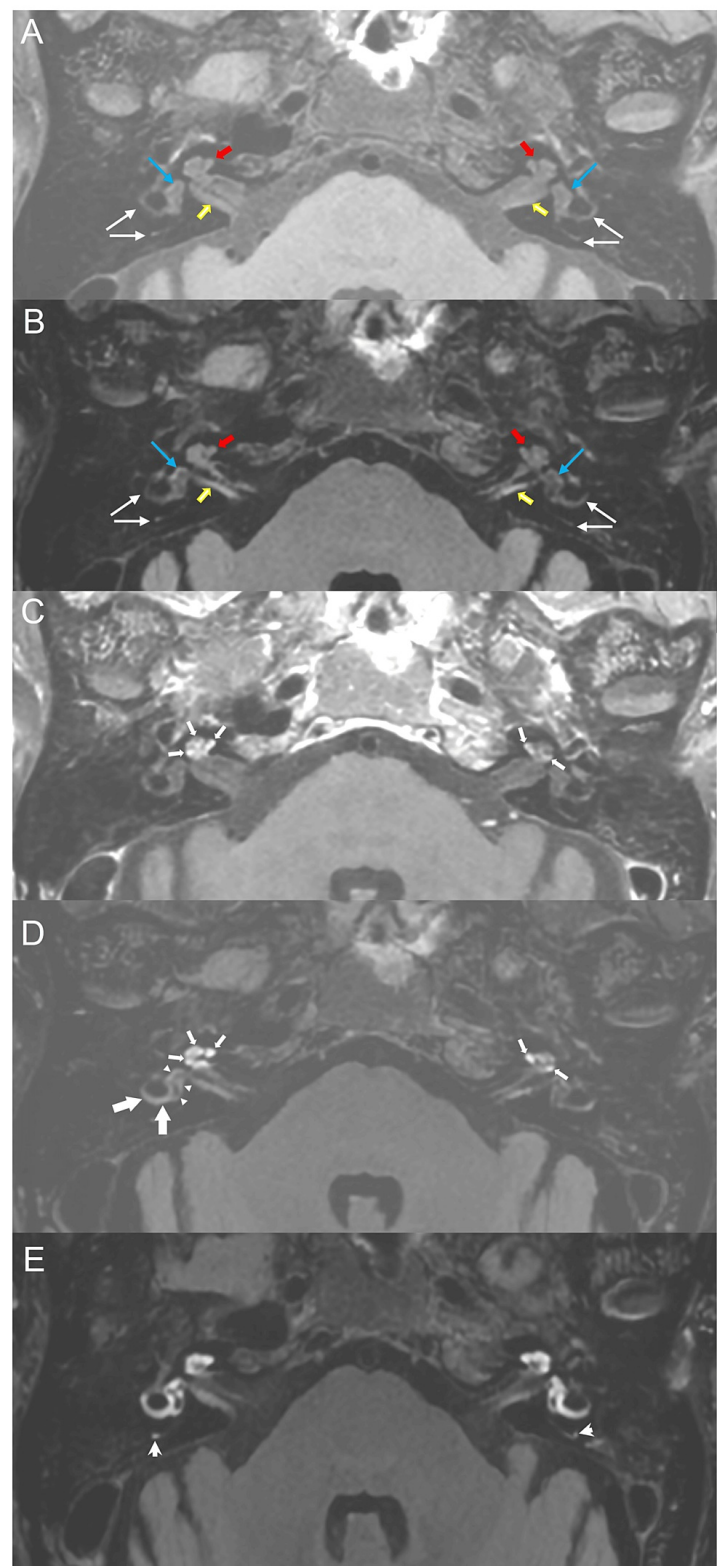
Internal auditory canal magnetic resonance imaging. Precontrast T1-weighted image (A) and precontrast three-dimensional fluid-attenuated inversion recovery (3D FLAIR) images (B) at the level of internal auditory canal (IAC, yellow arrows) show normal signal intensity of the bilateral cochlea (red arrows), vestibules (blue arrows) and semicircular canals (white arrows). After gadolinium-contrast enhancement, enhancement of the bilateral cochlea is noted (small arrows) on postcontrast T1-weighted images (C) and 10-minute-delay 3D FLAIR images (D). Subtle enhancement of the right vestibule (arrowheads) and right lateral semicircular canal (large arrows) is also noted on a 10-minute-delay 3D FLAIR image. Postcontrast, four-hour-delay 3D FLAIR image (E) shows profuse enhancement of the bilateral cochlea, vestibules and lateral semicircular and posterior semicircular canals (open arrow). In this patient, delayed high signal intensity in postcontrast 3D FLAIR image indicates the breakdown of blood-labyrinth barrier on both sides.
